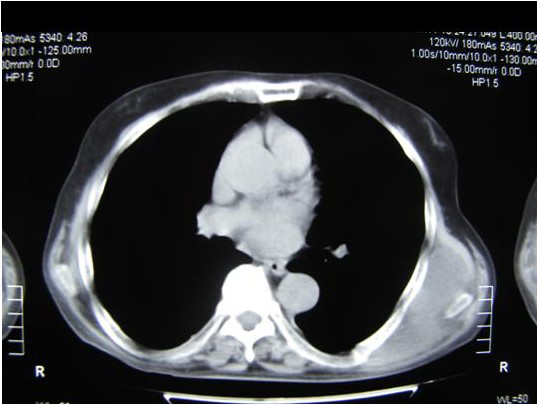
Computed Tomography of the patient showing the extrathoracic mass on the left posterior thoracic cage.
